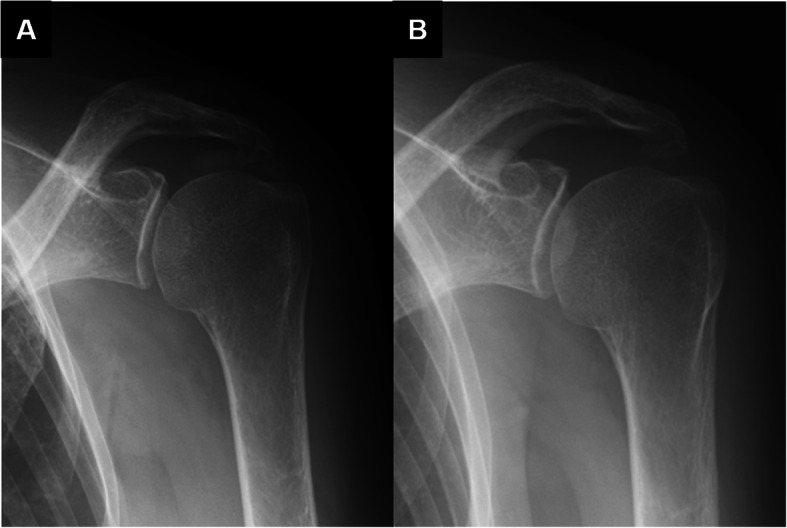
Plain radiographs of acute calcific tendinitis of the rotator cuff. (a) Plain radiography performed at the onset of acute calcific tendinitis shows calcium deposition in the rotator cuff in an 80-year-old woman. (b) After 3 weeks from the onset, calcium deposition has disappeared
